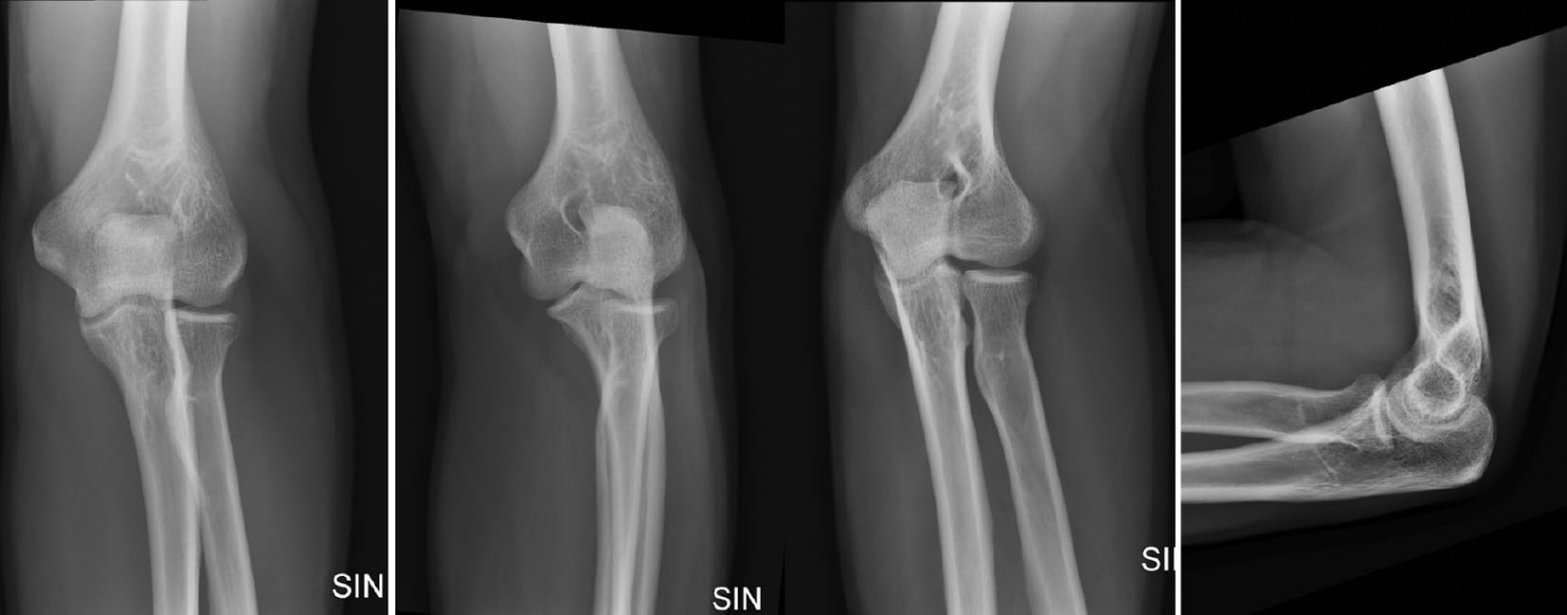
Example of normal adult radiographs including AP, external oblique, internal oblique and lateral projections used in this study.
